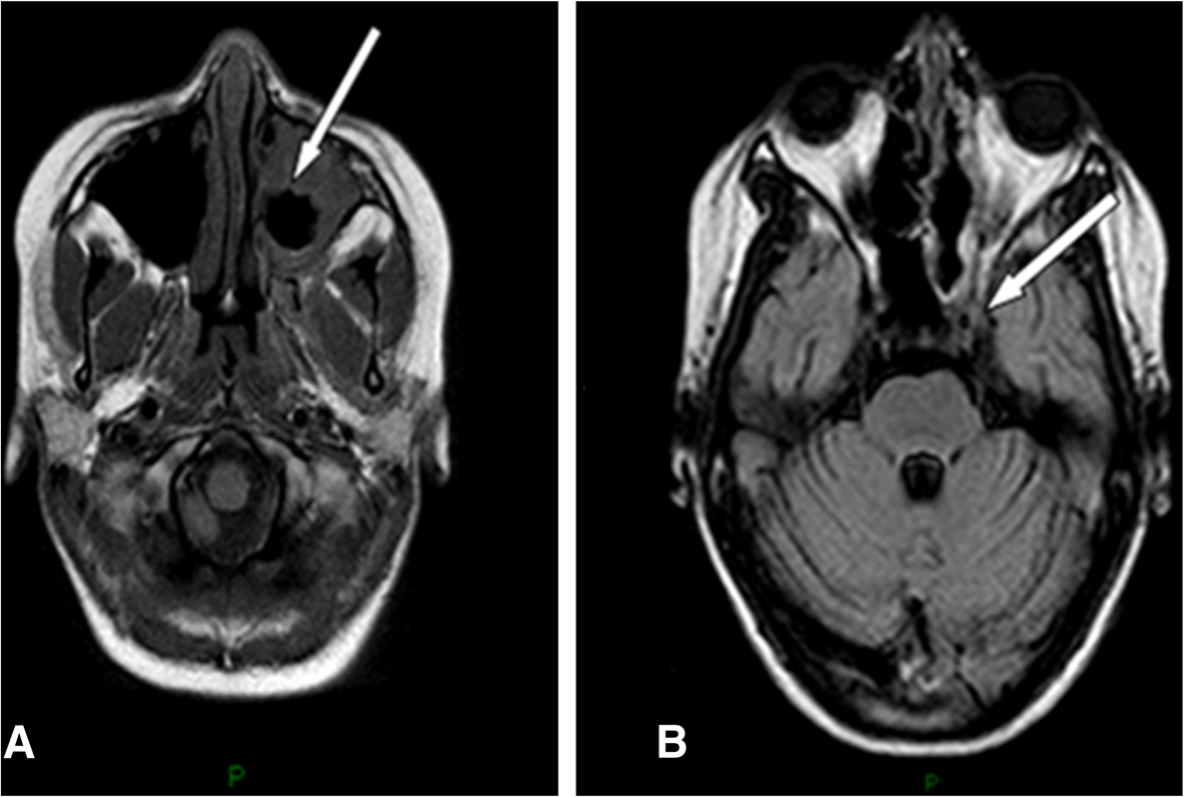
Magnetic resonance imaging of head. A. Postgadolinium T1-weighted axial magnetic resonance image showing nonenhancing tissue (arrow) in the left nasal cavity and periorbital site compatible with necrotic mucosa. B. Similar technique showing note semiocclusion of the left internal carotid artery (arrow) and thrombosis of the right cavernous sinus, although there was no radiographic evidence of cerebral infarction.
